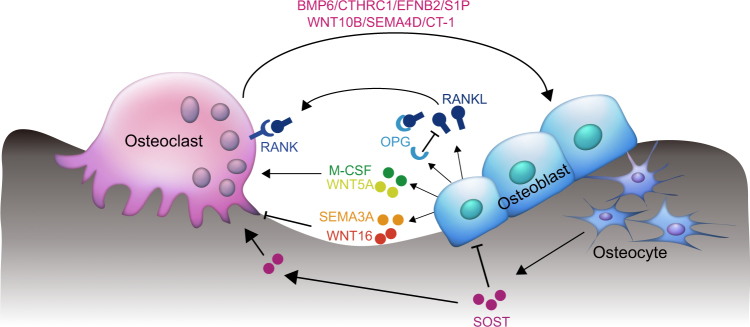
Paracrine actions of osteoblasts, osteocytes, and osteoclasts-derived factors. Molecules secreted by osteoblasts, osteocytes, and osteoclasts influence each other in a paracrine manner to maintain the balance of bone formation and bone resorption. Osteoblasts activate osteoclast formation by expressing M-CSF, RANKL, and WNT5A and inhibit osteoclast activity through OPG, a decoy receptor of RANKL, SEMA3A, and WNT16. Osteocyte-derived SOST inhibits osteoblast differentiation and stimulates osteoclastogenesis. Osteoclasts also secrete coupling factors such as BMP6, CTHRC1, EFNB2, S1P, WNT10B, SEMA4D, and CT-1 to act on osteoblasts and osteocytes and thereby influence bone formation.
